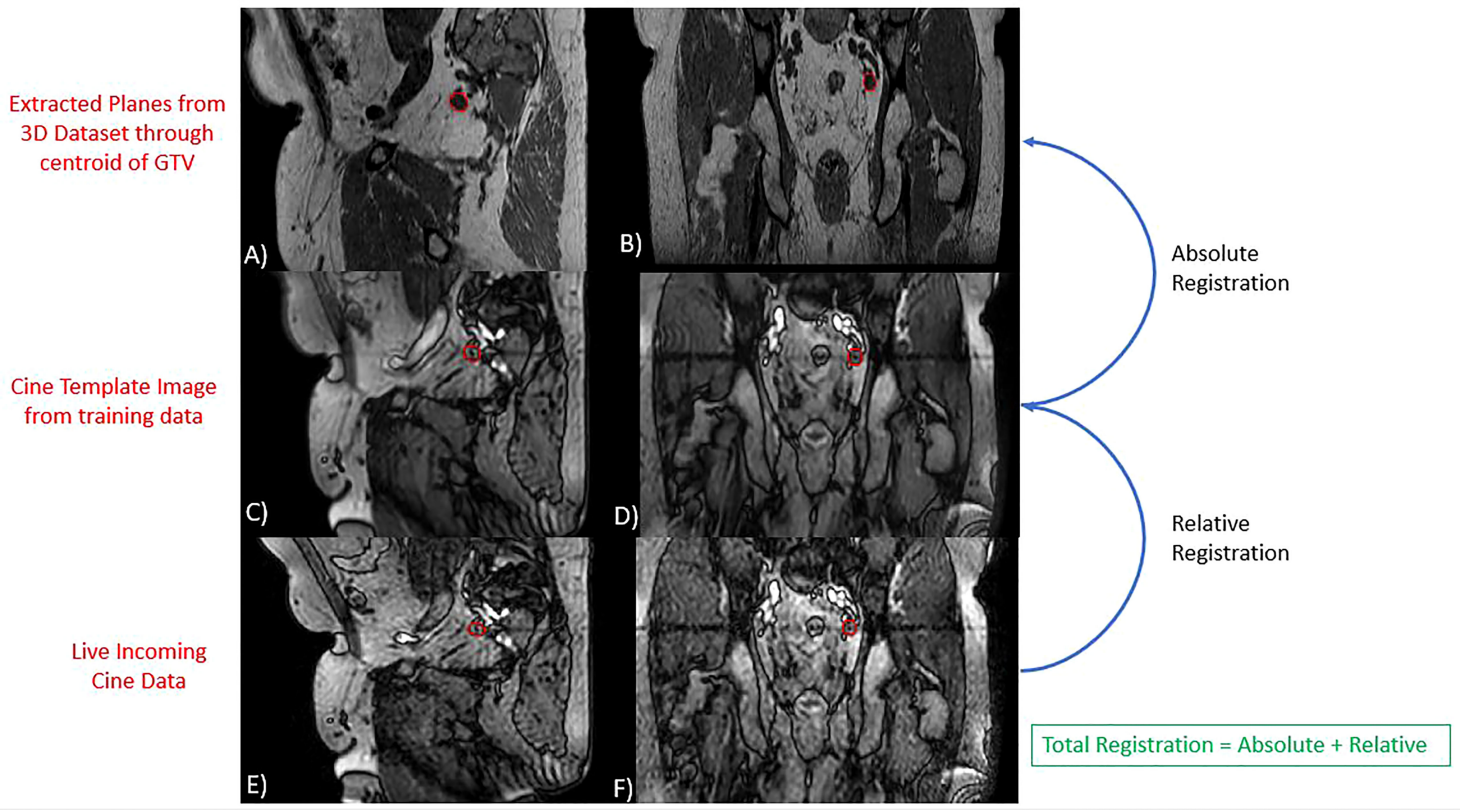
Overview of Tracking Algorithm. Images (A, B) represent extracted planes taken through the centroid of the GTV on the daily 3D MRI dataset. Images (C, D) are the sagittal and coronal template images generated from the algorithms training phase. Images (E, F) are live incoming sagittal and coronal cine motion monitoring images. In each image, the red contour represents the GTV structure contoured during planning (A, B) or identified by the tracking algorithm (C–F). The algorithms reported target position is equal to the sum of the relative and absolute registration components.
